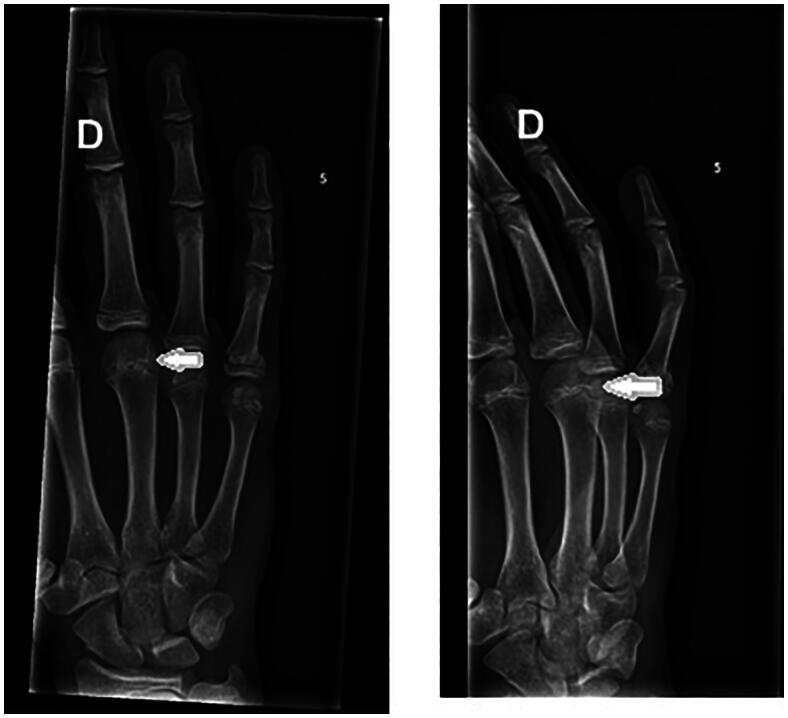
Radiograph July 2022, osteolysis of the third metacarpal head with sclerosis.
